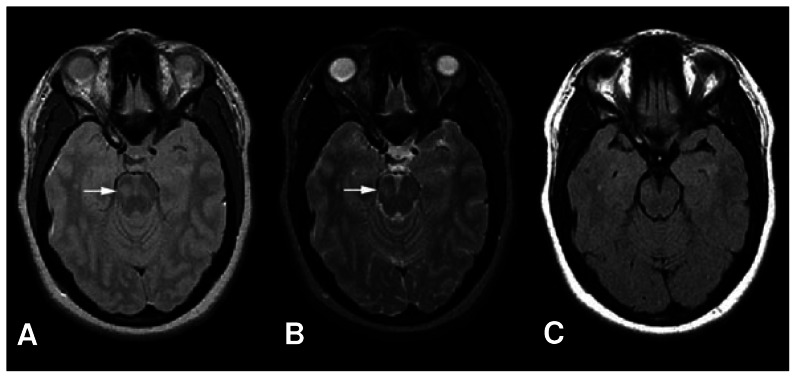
A typical Infratentorial lesion. (a) PD-w, (b) T2-w, (c) and FLAIR images of a patient with remitting relapsing multiple sclerosis (RRMS) demonstrate a pontine lesion (arrows) that is not demonstrated on the FLAIR sequence [2].
